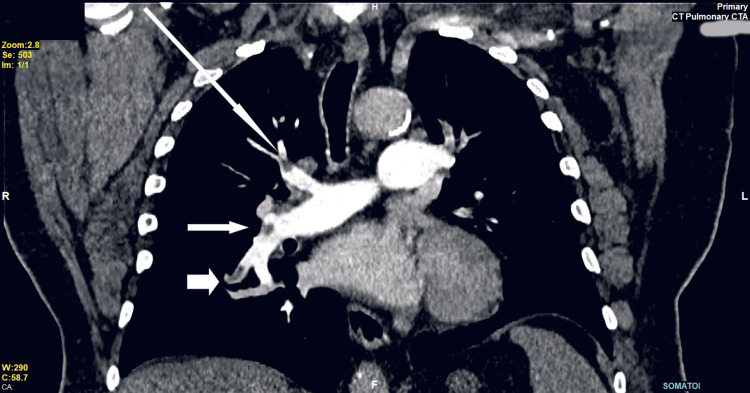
CT pulmonary angiogram, coronal view (Case 2). Arrows indicate pulmonary emboli.
